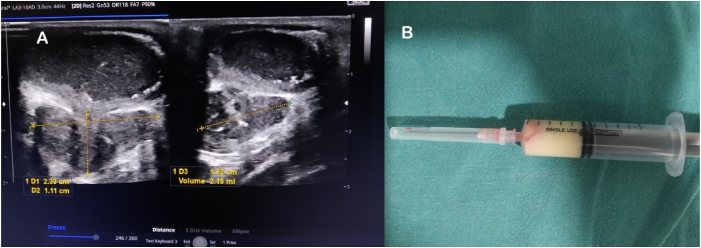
A. Ultrasound showing encapsulated lesion with iso to hyperechoic contents in the subcutaneous plane of the right axilla. B. Diagnostic aspiration showing thick pus.
